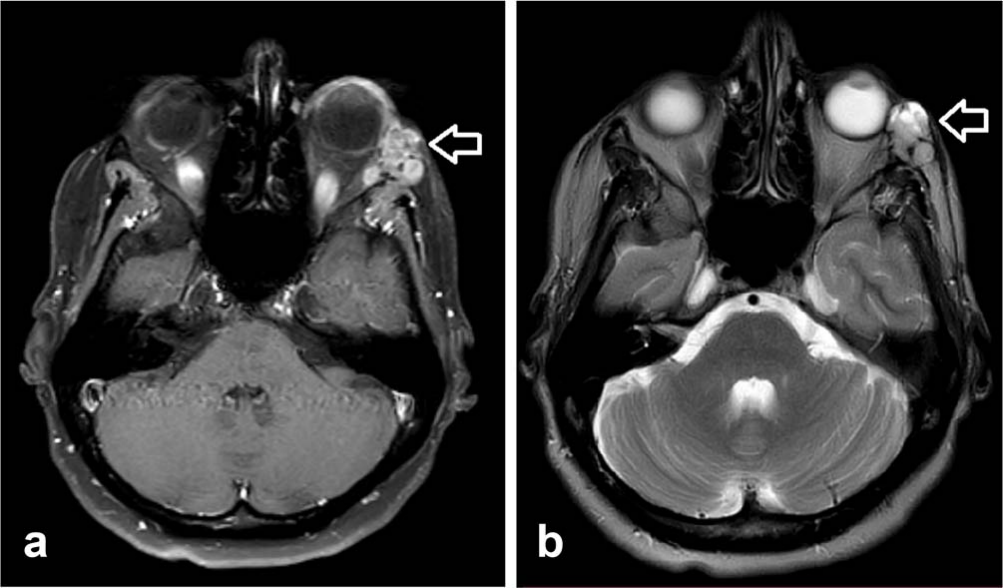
Orbit MRI showed lobulated well-defined lesion, hyperintense to intermediate on T2W (a), hypointense on T1W (b) structures with cystic changes measuring 2 × 1.5 cm and 2 × 1.2 cm. After IV contrast administration they showed heterogeneous moderate enhancement.
